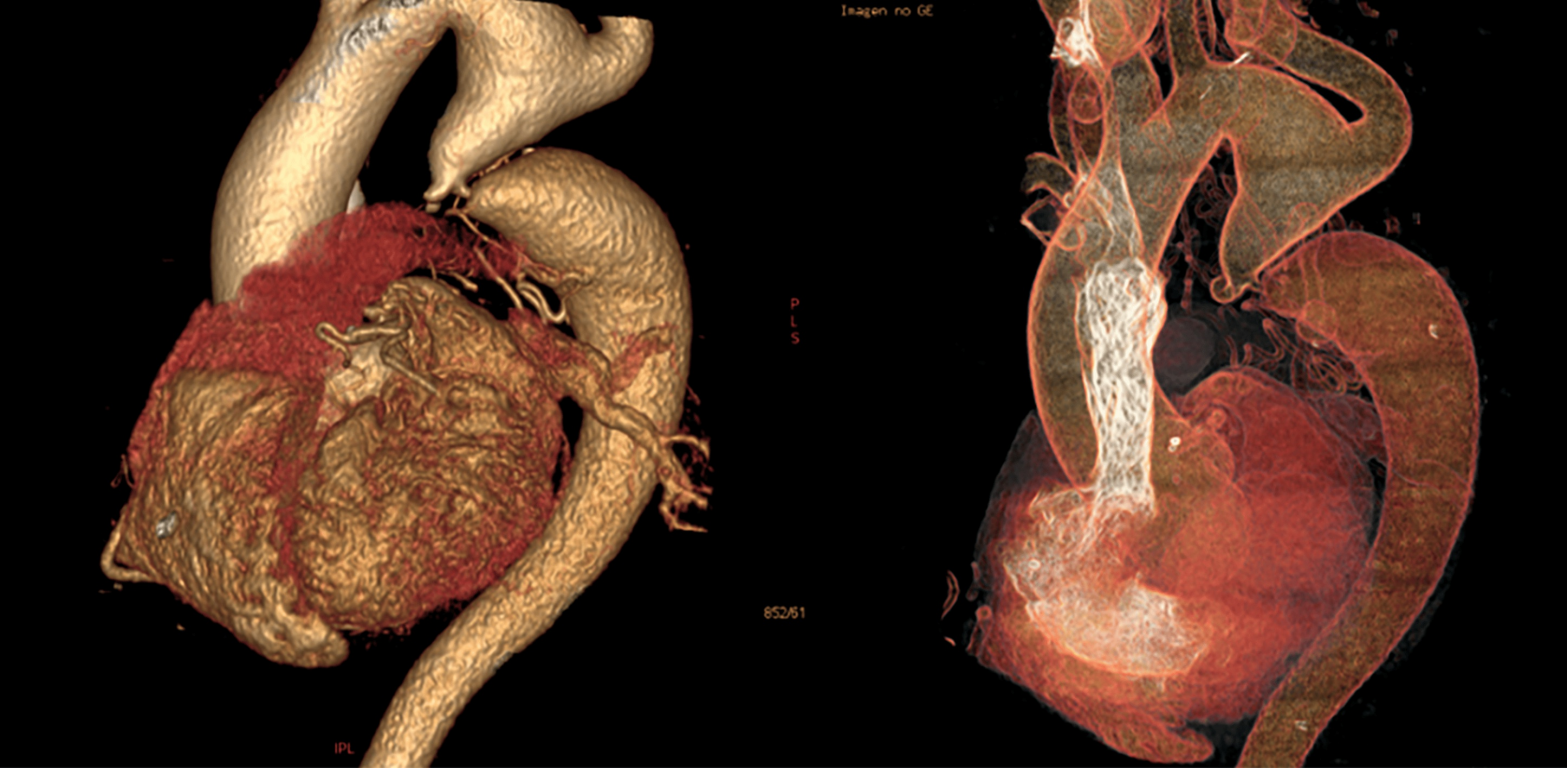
Three-dimensional reconstructions from aortic CTA demonstrating a postductal aortic coarctation with significant dilatation of the aortic segments proximal and distal to the narrowing. CTA: CT angiography
